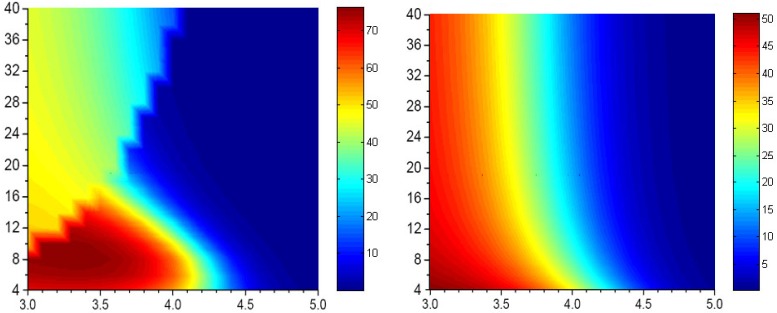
Contour polts of the CT exciton (in percentage) as a function of interchain distance (x axis) and chain size (y axis) of the first (right) and second (left) excited states in polyene dimers calculated using model 1 [46]. Reproduced with permission from American Chemical Society.
